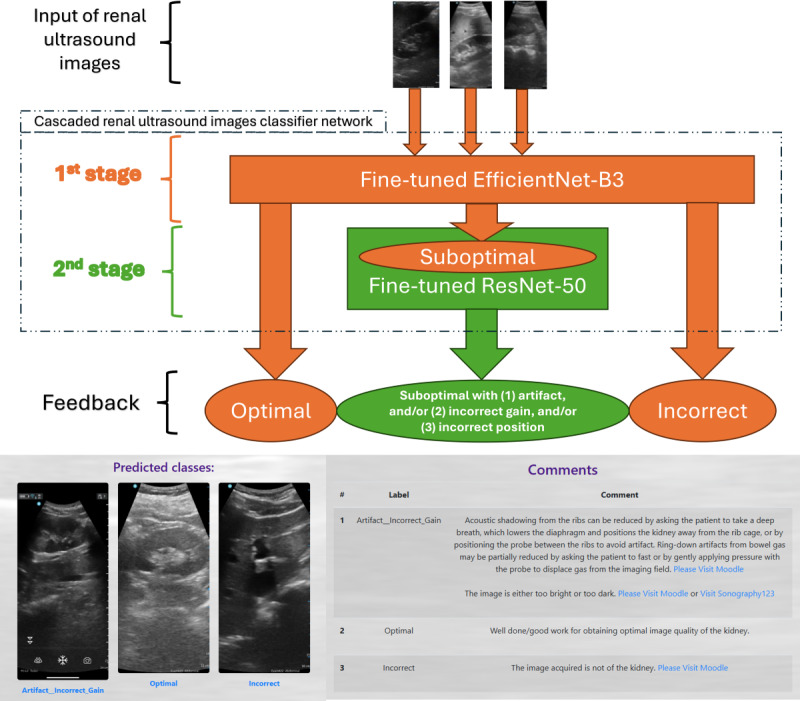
Cascaded renal ultrasound image grading system with automated grading and feedback for students (demonstration video in Multimedia Appendix 1).
